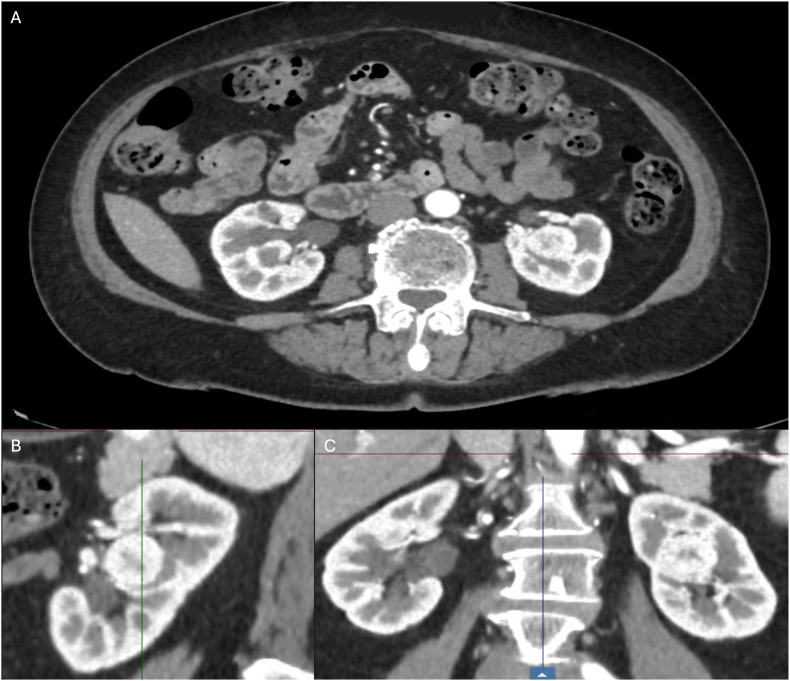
Arterial phase contrast-enhanced CT images of the left kidney showing a completely endophytic renal mass in axial (A), sagittal (B), and coronal (C) planes.
